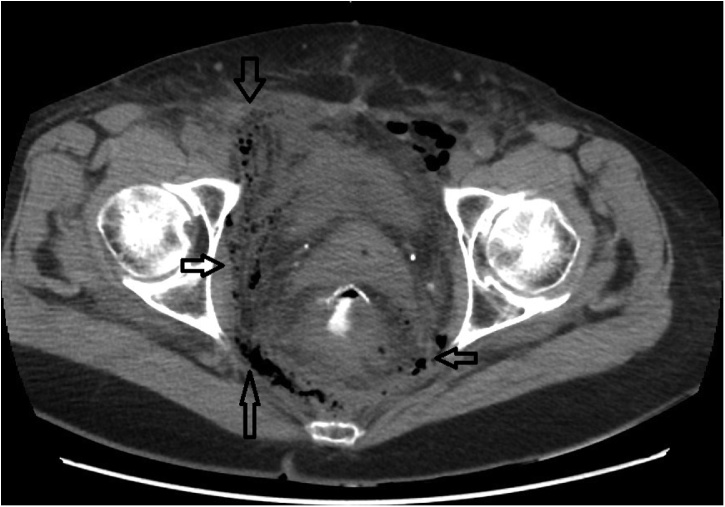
CT scan of an anastomotic leak and infection of the soft tissue in the right lower abdomen.
